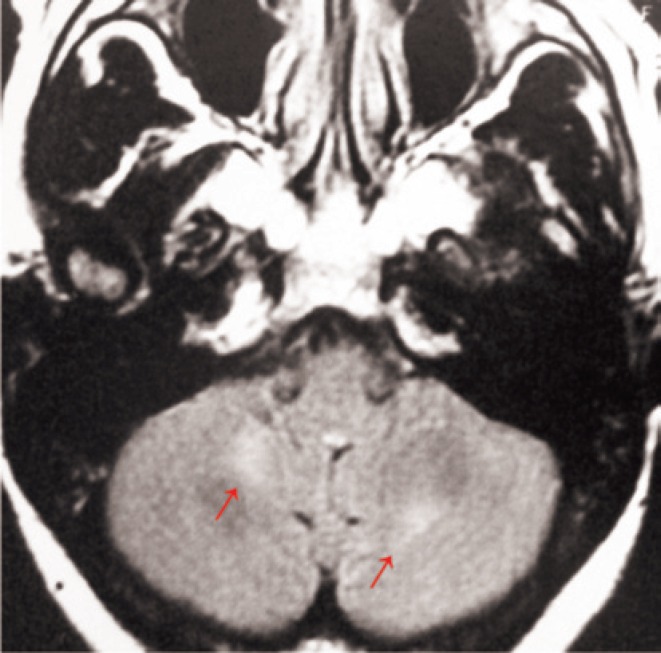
Contrasted T1-weighted magnetic resonance imaging sequence showing nonspecific hyperintensities in cerebellar hemispheres (red arrows)
